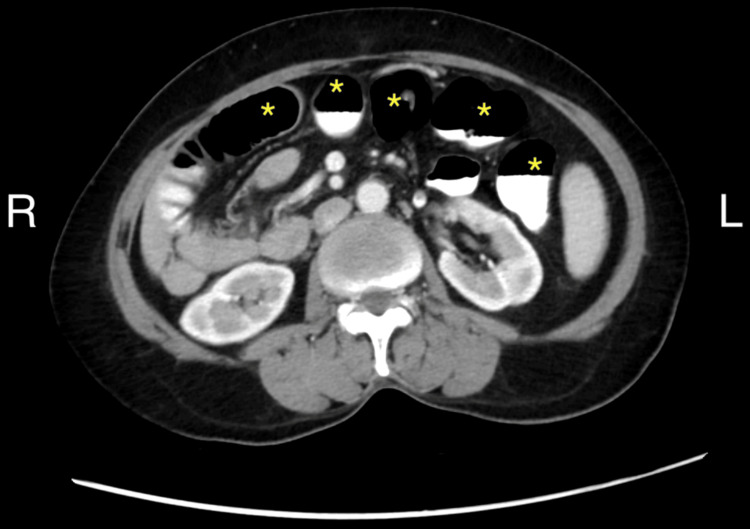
Axial contrast-enhanced CT image showing multiple fluid-filled, dilated bowel loops (marked with *) without evidence of midgut volvulus (absence of the whirl sign)
